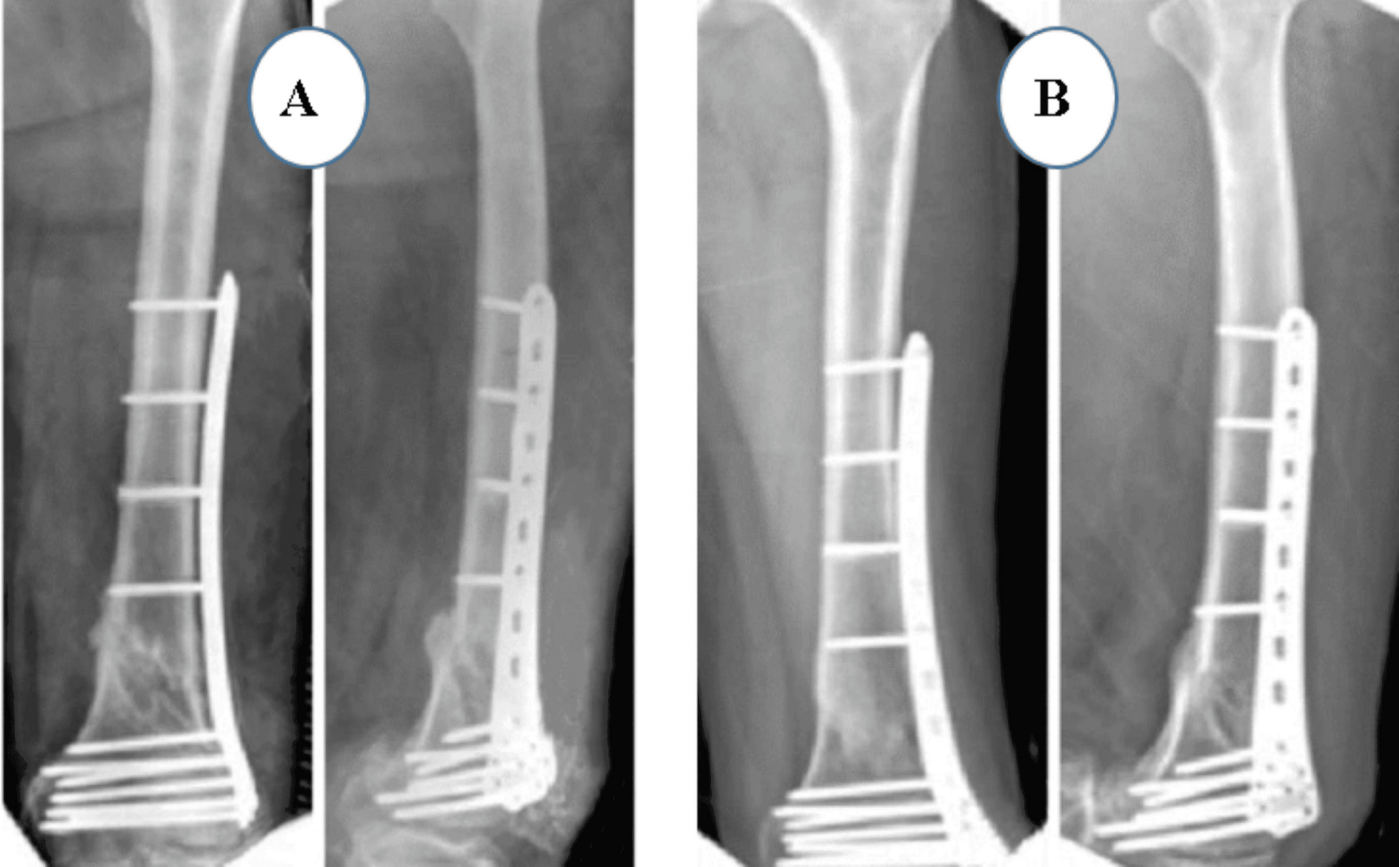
Case 2 follow-up X-rays at (A) three and (B) six months
